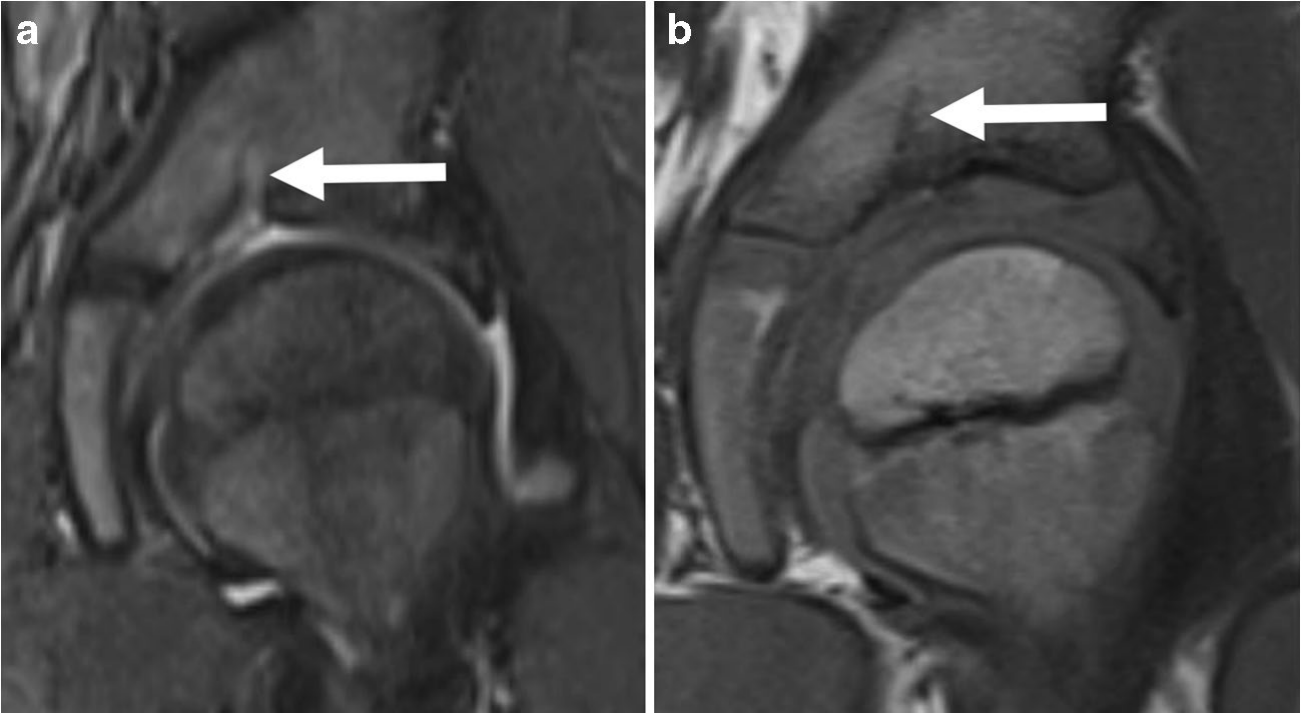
Five-year-old male. Coronal short tau inversion recovery (a) and coronal T1-weighted (b) MR images show fluid-like findings within the SARN (arrows) of the left hip, classified as a SARN type-1
